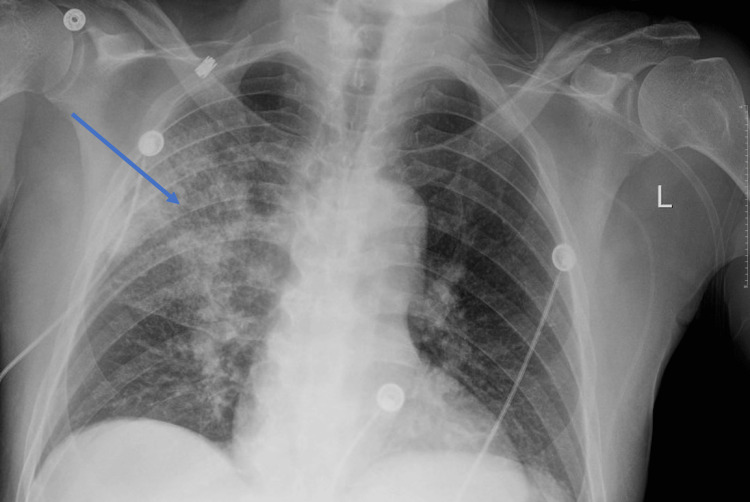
Chest X-ray shows the presence of a right upper lobe consolidation, consistent with pneumonia
